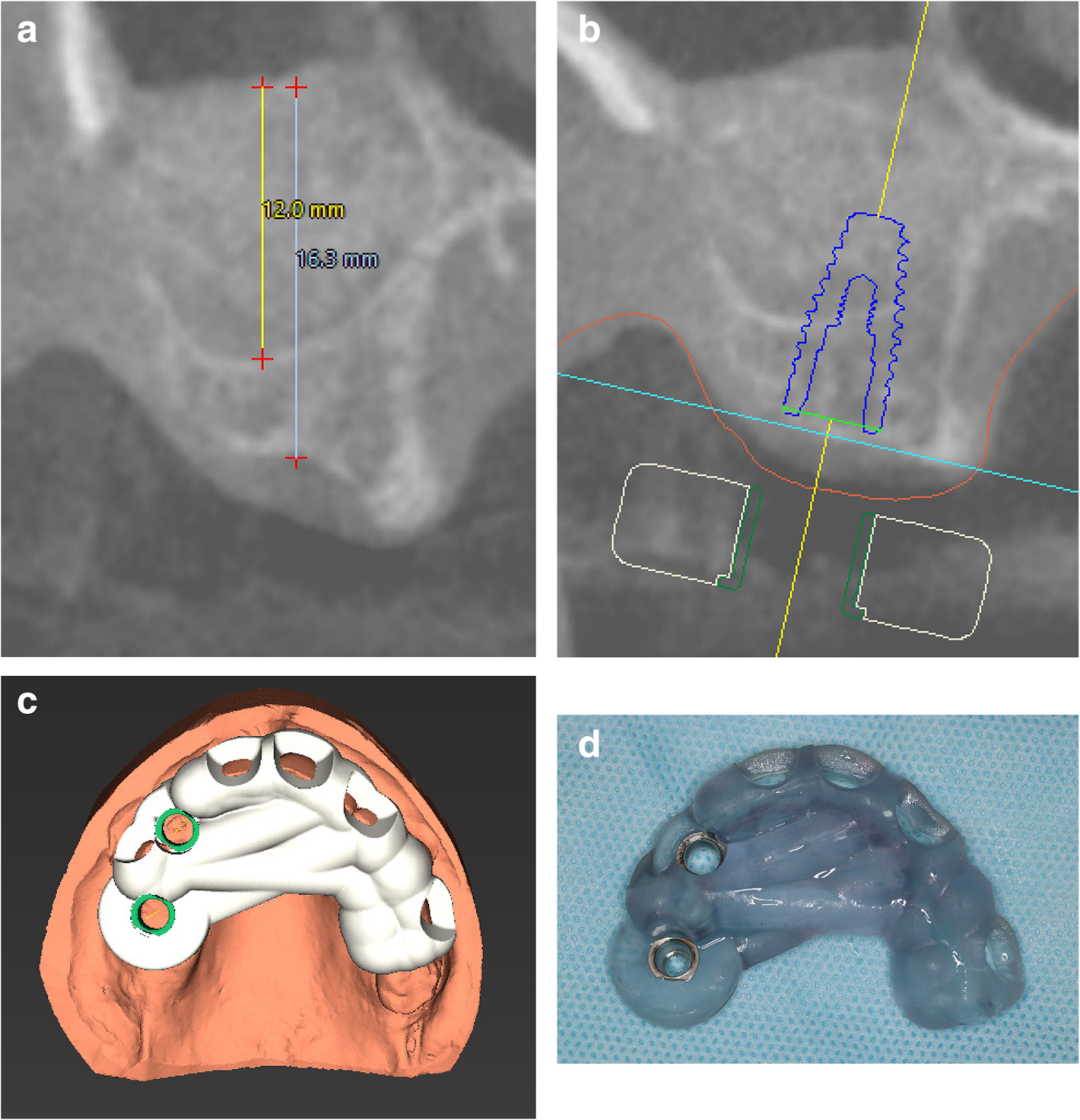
a 14 weeks follow-up CBCT to plan for implant placement. b Planning implant position into the grafted sinus using coDiagnosticX software. c Digital design for the surgical guide. d 3D-printed surgical guide
